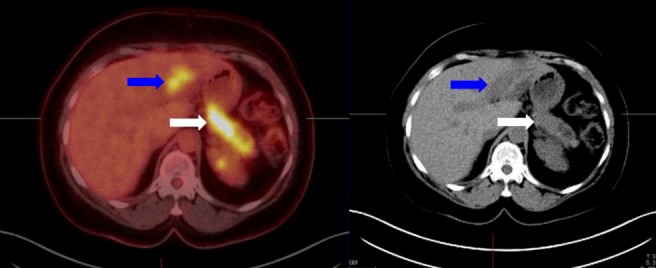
Follow-up PET/CT scan There is a new FDG-avid lesion in the left hepatic lobe on the PET axial images *(blue arrow, left image) *with a corresponding hypodense correlate on the CT axial image *(blue arrow, left image)*. Also seen again the original FDG-avid pancreatic tail lesion *(white arrow).*
